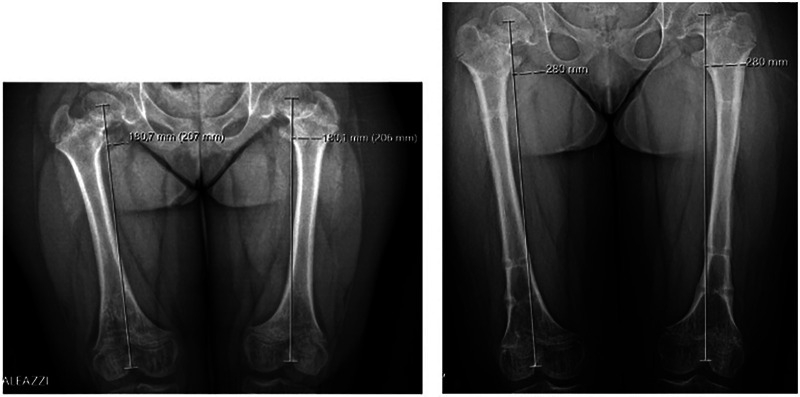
Radiographic measurements of the femur before surgery (left image) and after removal of external fixators (right image).
